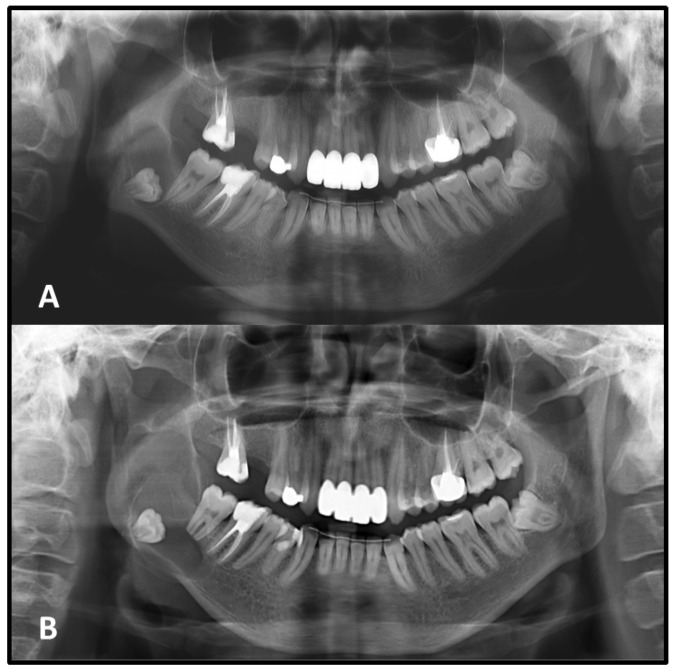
A unilocular radiolucent lesion with well-defined borders associated with an impacted right mandibular third molar observed on the initial panoramic radiograph. The lesion demonstrates mild cortical expansion, is in proximity to the inferior alveolar canal, and has caused displacement of the impacted tooth (A) The same lesion demonstrated considerable growth over the three-year interval, with displacement of the impacted third molar toward the inferior mandibular border, extension into the inferior alveolar canal, and associated root resorption of the adjacent second molar. Marked cortical expansion, thinning, and cortical destruction were also observed (B).
